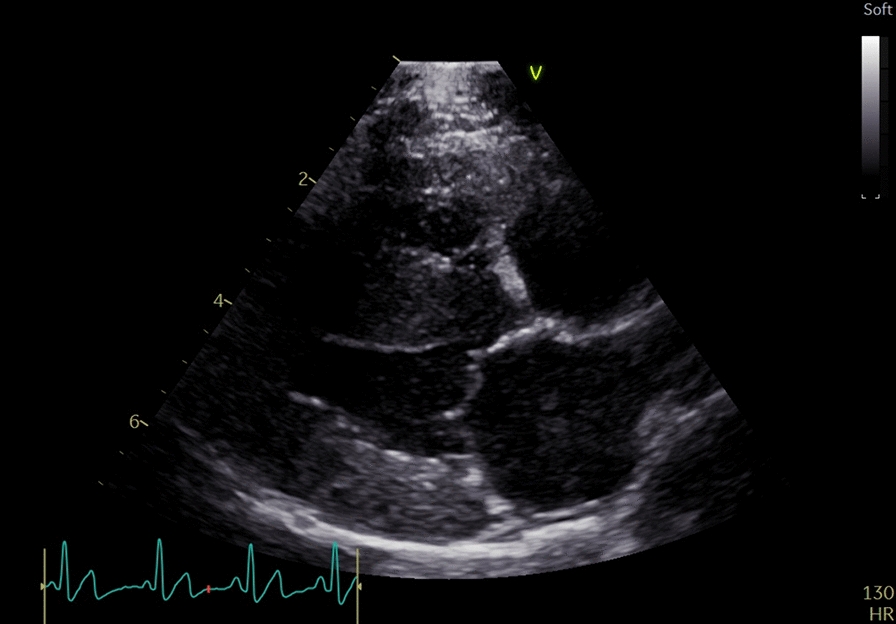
Echocardiography performed one month after commencement of treatment from the right parasternal long axis four chamber view showing normal cardiac morphology
